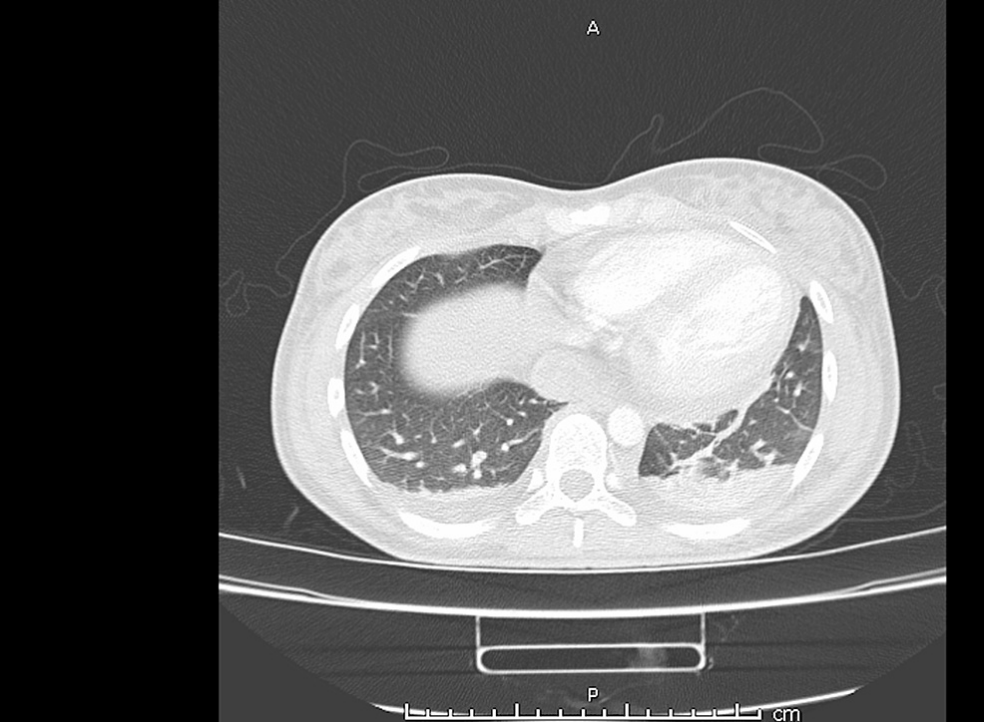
CT chest in the pulmonary window showing bilateral pleural effusion
